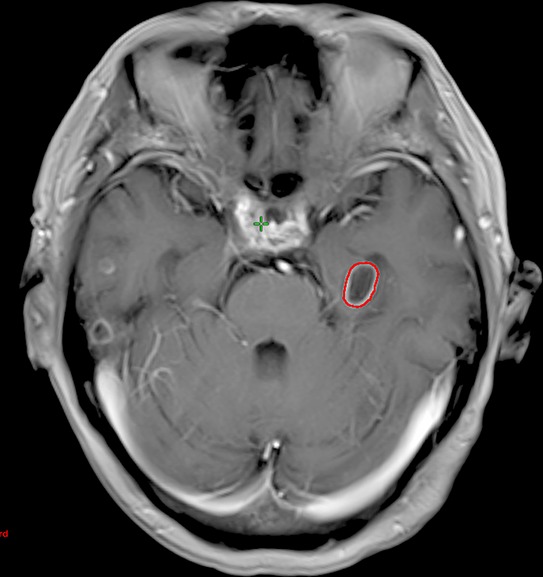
Magnetic resonance image of a patient who has a hippocampal metastasis The red contour represents the hippocampal metastasis.
